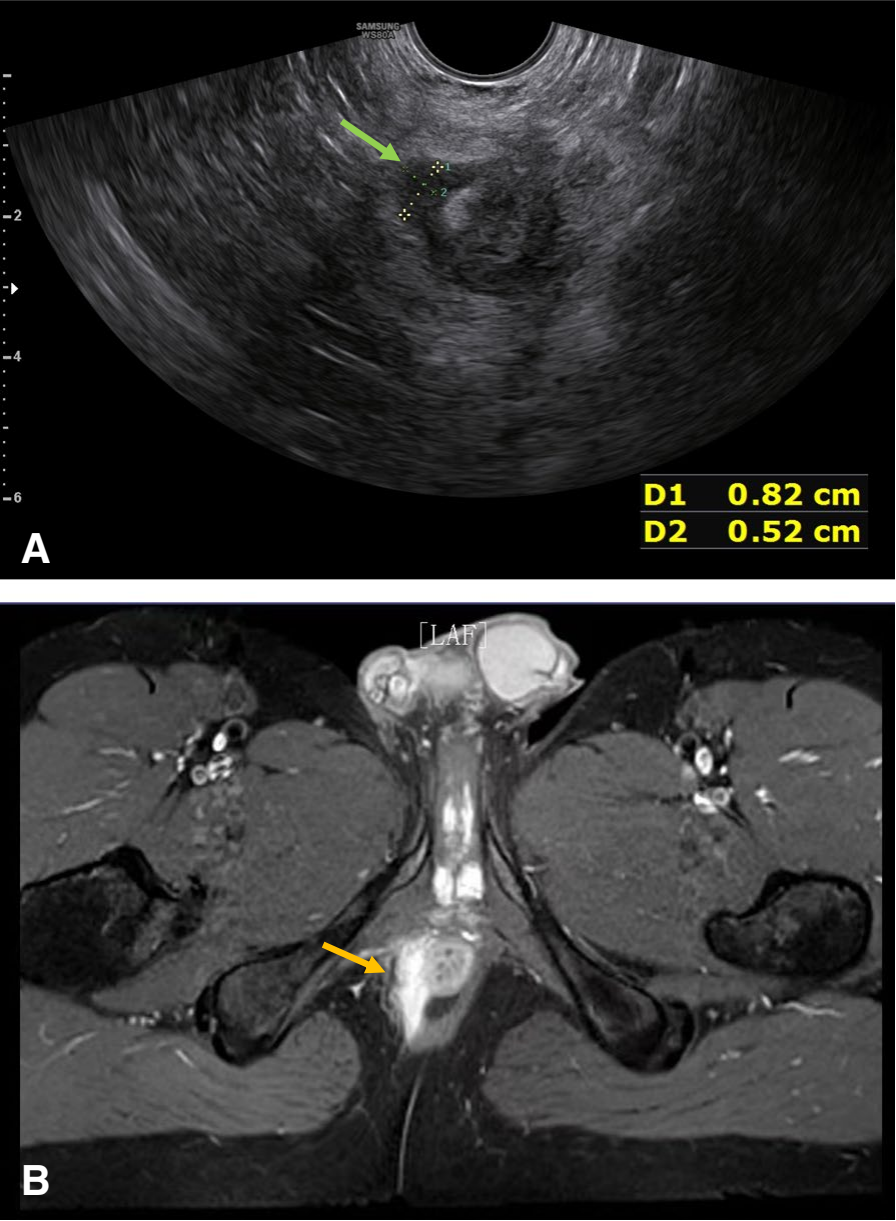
Anal fistula type III in pelvic 3D US and MRI images. A Pelvic 3D US image of a anal fistula(green arrow); (B)MRI image of a anal fistula(orange arrow)
